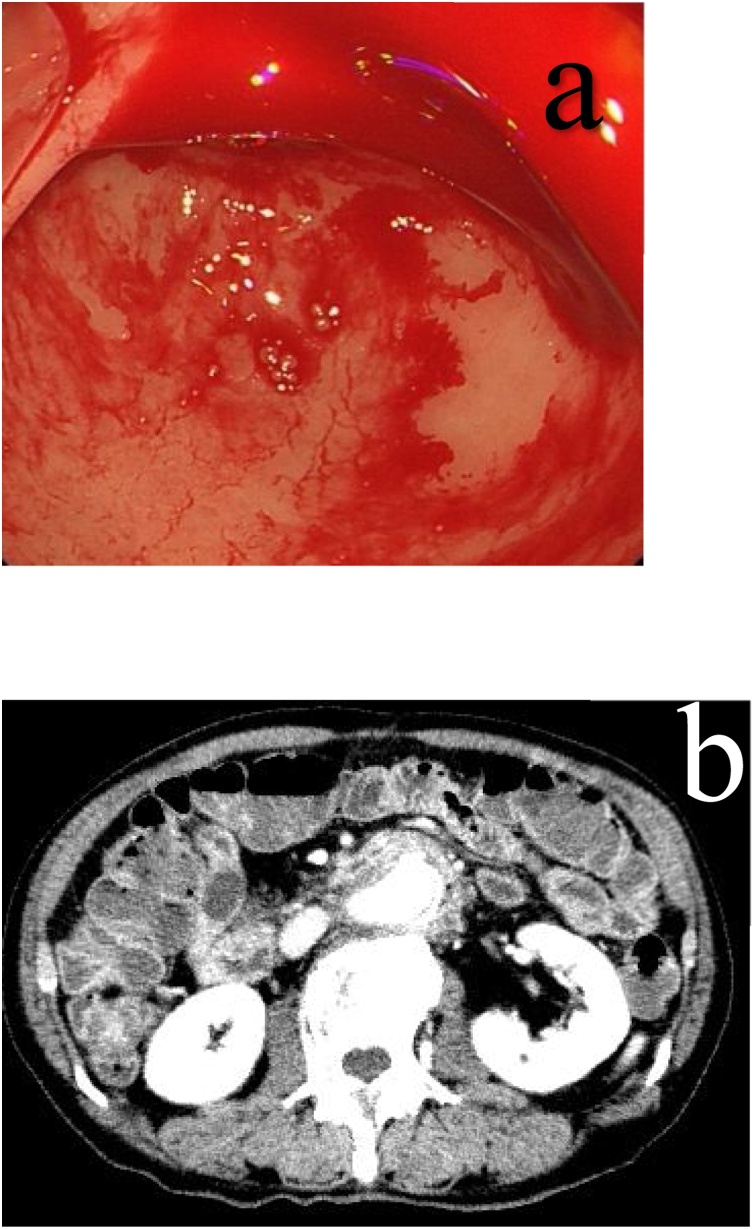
a. Endoscopy of the upper gastrointestinal tract shows the reflux of a large quantity of blood in the stomach from the duodenum. b. CT scan shows hyperplasia of the soft tissue and air bubbles around the artificial blood vessel.
